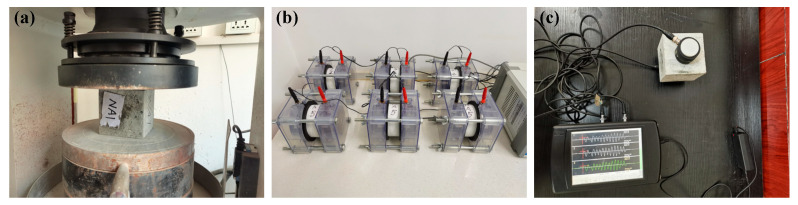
(a) Compressive strength; (b) Coulomb electric flux; (c) ultrasonic wave velocity.
